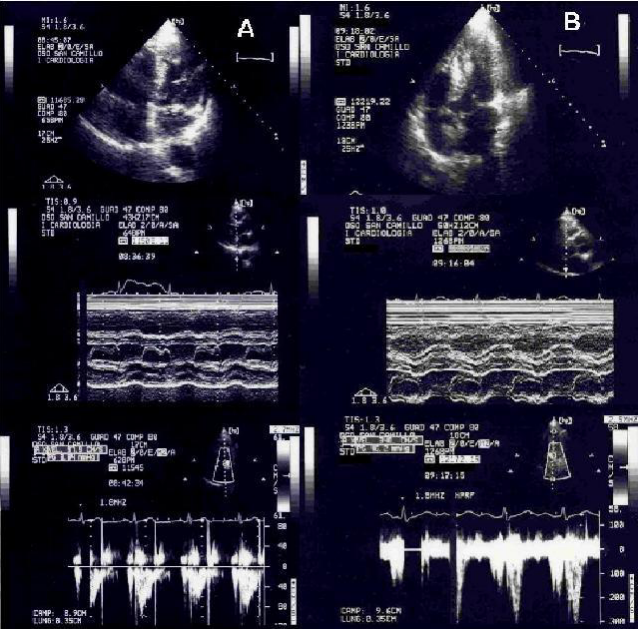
Rest and Dobutamine echocardiogram following mitral valve repair. Panel A: Top: Two-dimensional echo, apical 4-chamber view: normal coaptation of mitral leaflets; systolic anterior movement (SAM) is absent. Middle: M-Mode echo, mitral valve: normal coaptation of mitral leaflets. Bottom: Two-dimensional echo, PW Doppler in left outflow tract (LVOT):normal peak systolic velocity. Panel B: Top: Two-dimensional echo, apical 4-chamber view: SAM of anterior mitral leaflet is present. Middle: M-Mode echo, mitral valve; SAM is present. Bottom: Two-dimensional echo, PW Doppler in LVOT: elevated peak mid-systolic velocity corresponding to 46 mmHg outflow gradient.
